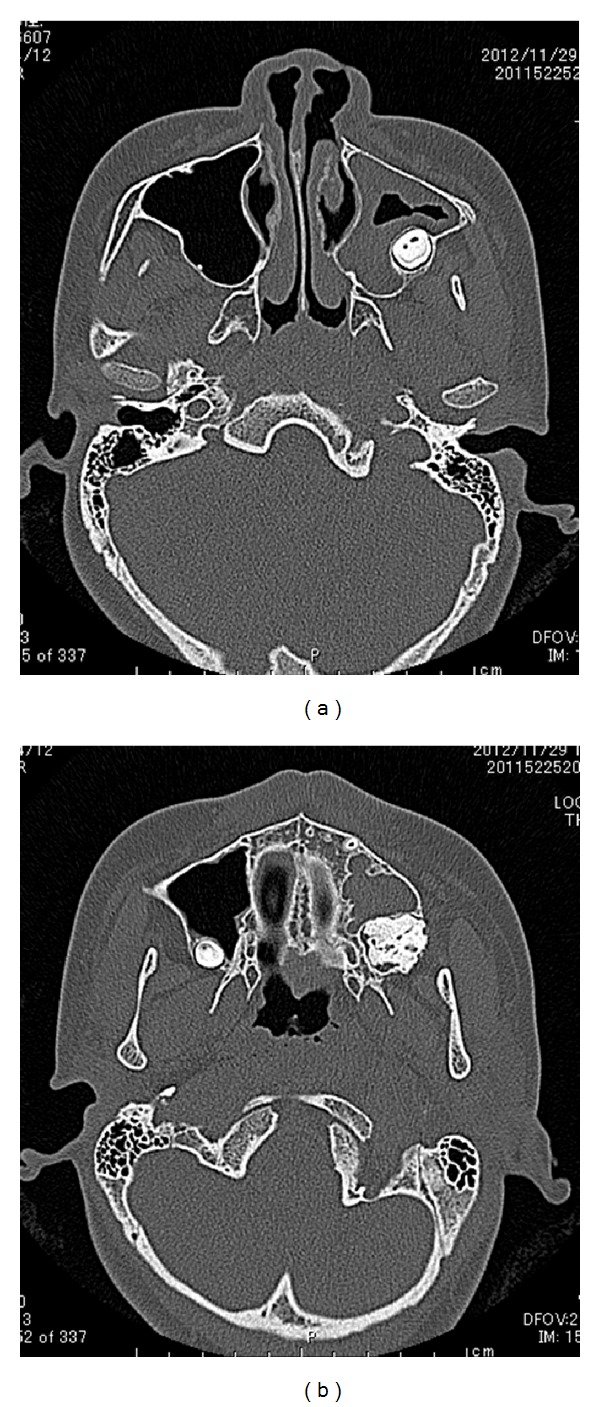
Axial CT view of the lesion.
